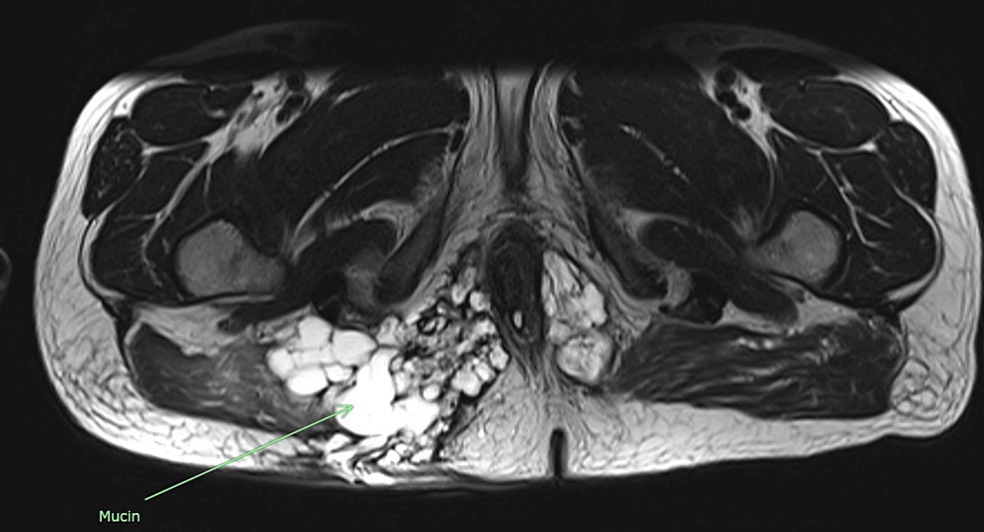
The MRI (coronal section) showed a complex, multiloculated collection with a complex fistula seen communicating with the right buttock.
